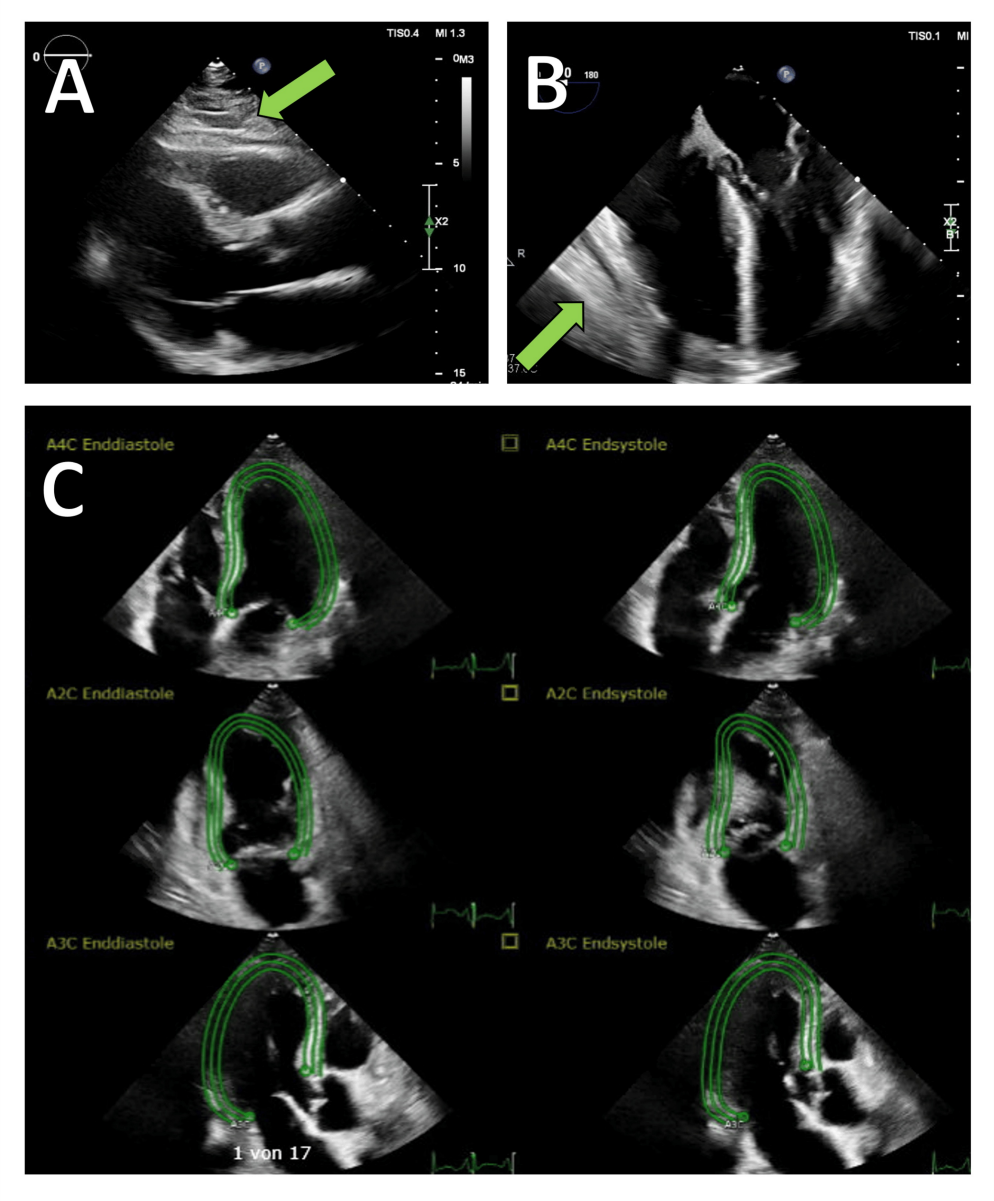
Echocardiographic assessment of constrictive pericarditis. (A) Long-axis transthoracic echocardiographic view showing pericardial thickening (arrow). (B) Transesophageal four-chamber view highlighting the constrictive pericardium (arrow). (C) Doppler evaluation demonstrating a restrictive filling pattern.
